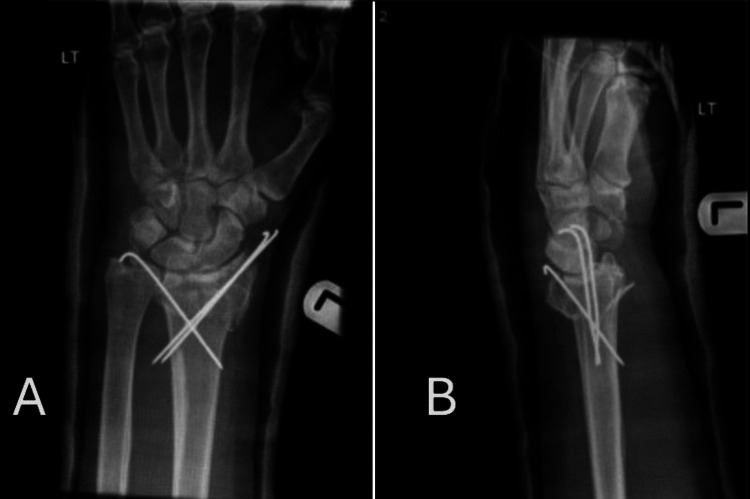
Anteroposterior (AP) and lateral views of the wrist X-ray with K-wire fixation. A: Anteroposterior (AP) view of wrist X-ray with K-wire fixation. B: Lateral view of the wrist X-ray with plate fixation.
